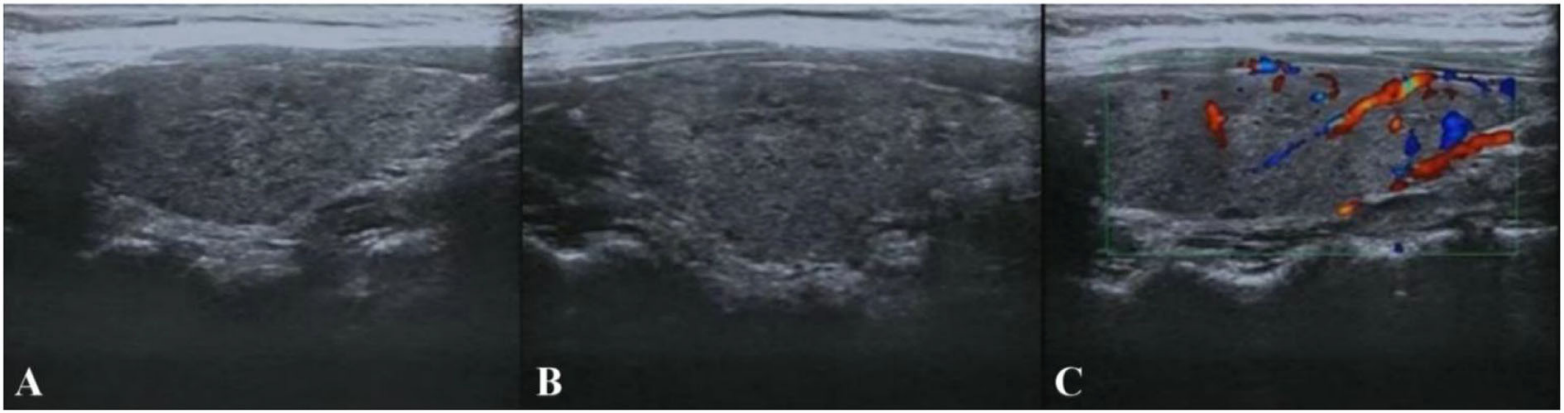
Thyroid color ultrasound. (A,B) The size and morphology of the left and right lobes of the thyroid gland were normal, the thickness of the isthmus was normal, the border was clear, the envelope was intact, the internal echogenicity was diffuse rough and heterogeneous, lamellar hypoechogenicity was seen. (C) No abnormal flow signals were seen.
